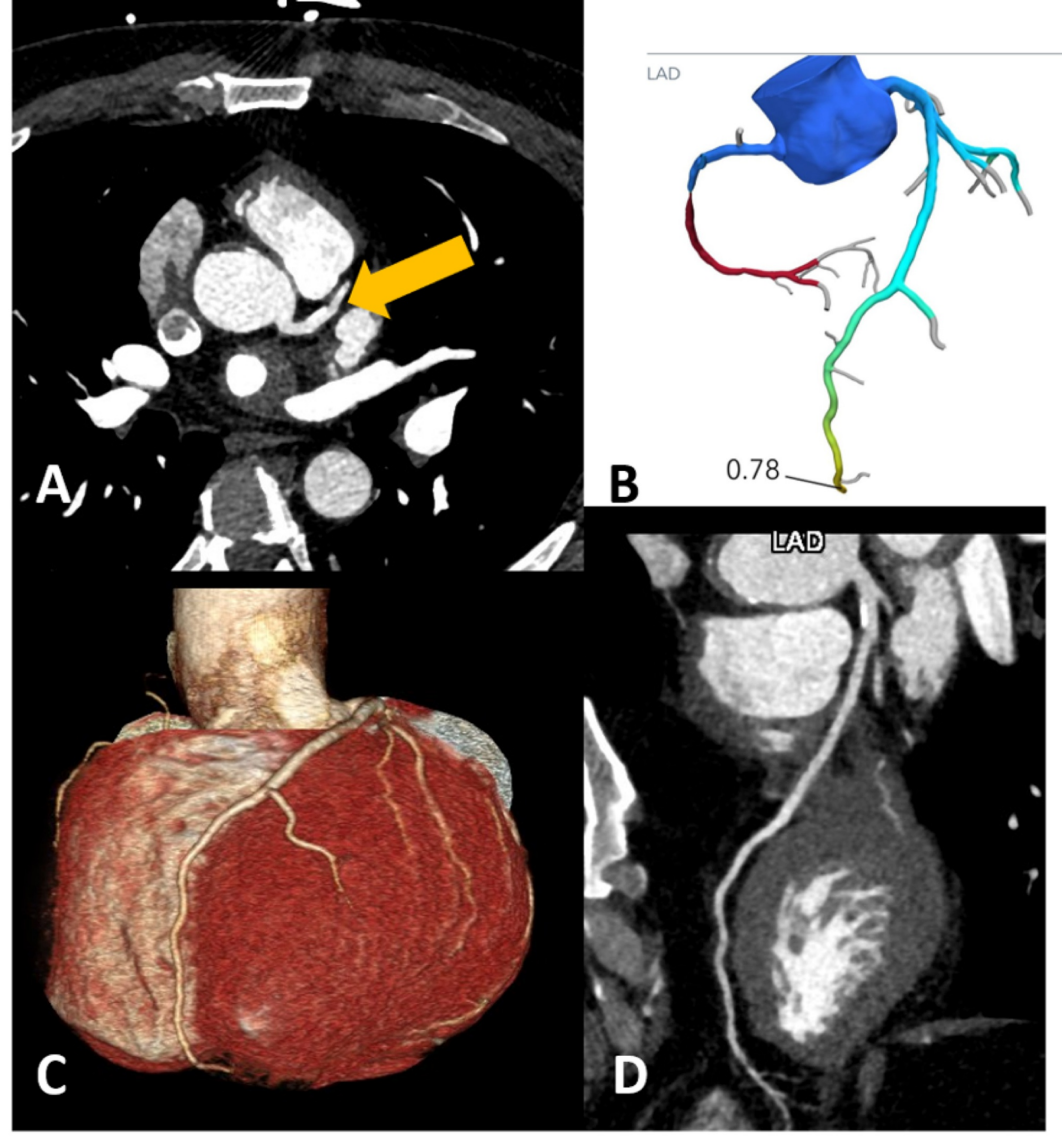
CCTA without cardiac motion, respiratory motion, and with properly dilated coronary arteries. (A) CCTA with properly opacified and dilated the left main coronary artery and LAD (yellow arrow). (B) Successful FFRCT analysis performed with values demonstrated for the LAD. (C) Three-dimensional reconstruction of the heart with a well-visualized LAD. (D) Virtual reformation of the LAD with minimal proximal stenosis from a calcified plaque. CCTA, coronary CT angiography; FFRCT, fractional flow reserve derived from CT angiography; LAD, left anterior descending artery
